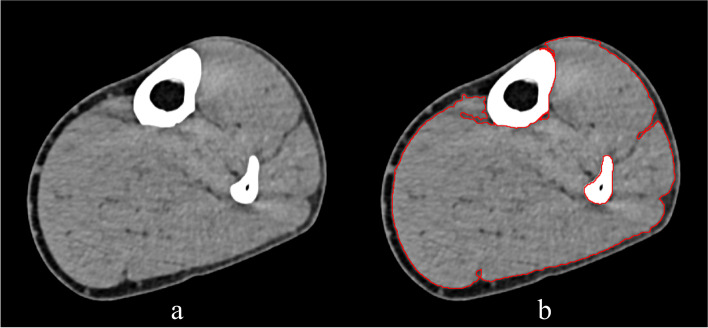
Lower leg CT plain scan and muscle area marking. a CT cross-section image of the lower leg (10 cm below the tibial tubercle). b Muscle boundary was marked with ImageJ; the cross-sectional area of the muscle was automatically calculated
